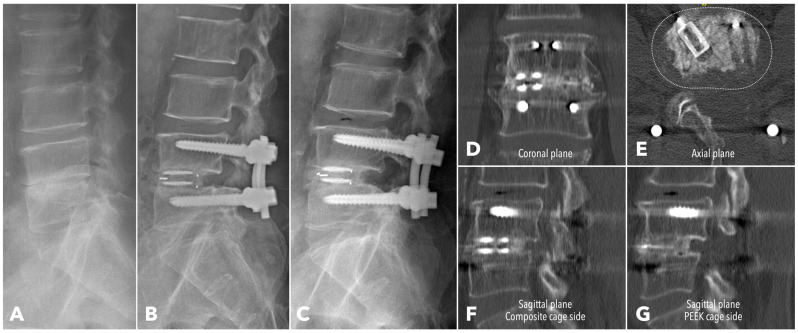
A 62-year-old male patient underwent BETLIF at L4-5 due to painful disc degeneration with restoration of the disc height after the surgery (A,B). The postoperative 6-month X-ray showed consolidation of the bone graft (C). The 1-year CT scan revealed Bridwell grade I solid fusion with remodeling of the bone graft at both coronal plane (D) and sagittal plane (F,G) reconstructions. The axial plane reconstruction (E) demonstrated the cage footprint and the fusion bed. The white dashed line indicates the margin of the vertebral body.
